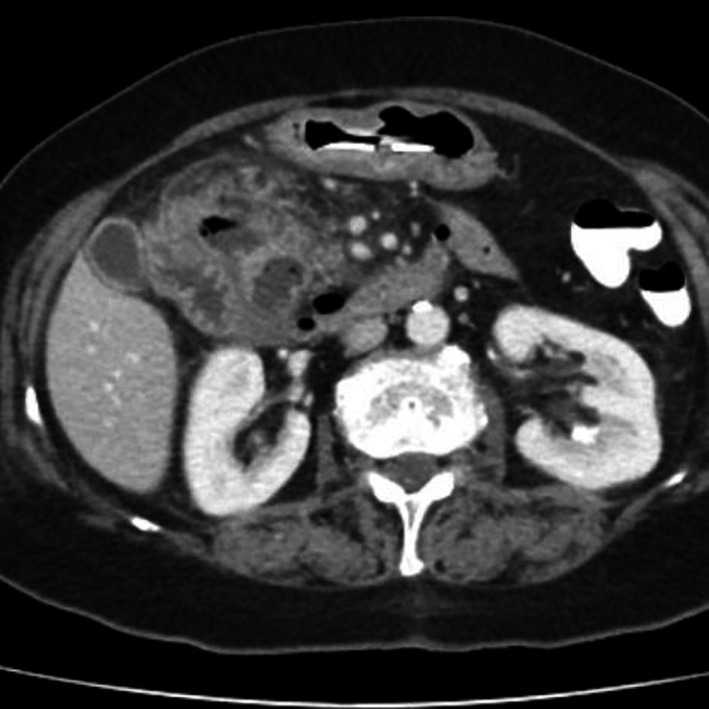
CT revealed a couple of ill‐defined and abscess‐like lesions interposed between duodenum and pancreas along with pancreatic head enlargement and peripancreatic inflammatory changes
